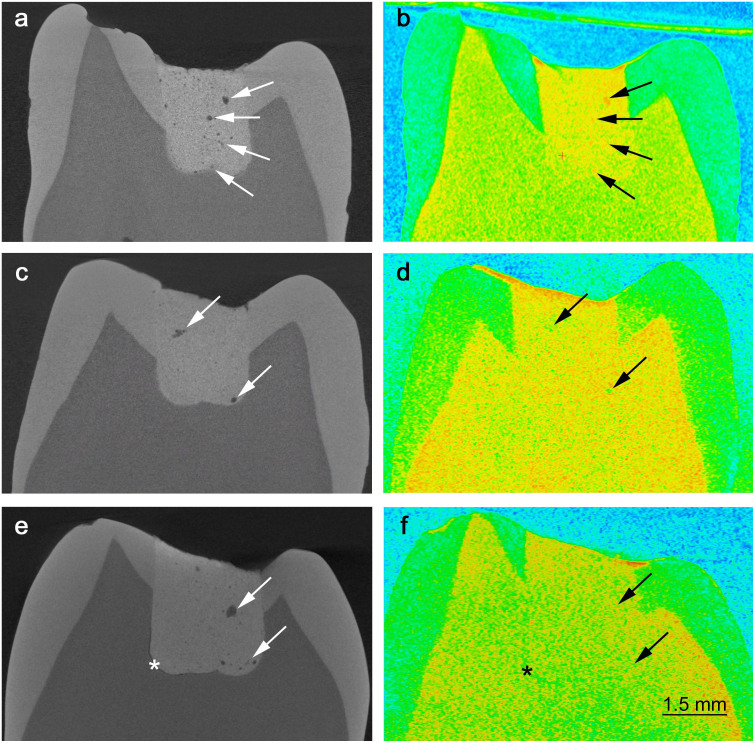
Extracted teeth restored with GIC. Pores and cracks are better visible in the X-ray images due to better resolution (a, c, e). Poor adaptation (*) of the more viscous restorative cement (Poly) at the bottom of the cavity is observed (e), while this problem is less evident for the less viscous cement (a, c). The presence of liquid inside or adhered to the internal walls of some of the larger pores is evident (in red, due to the higher attenuation coefficient of hydrogen) in the neutron image (b). The neutron images also suggest that interconnecting pores or cracks are filled with liquid (b, f), while some of the larger pores seem to be empty (d).
